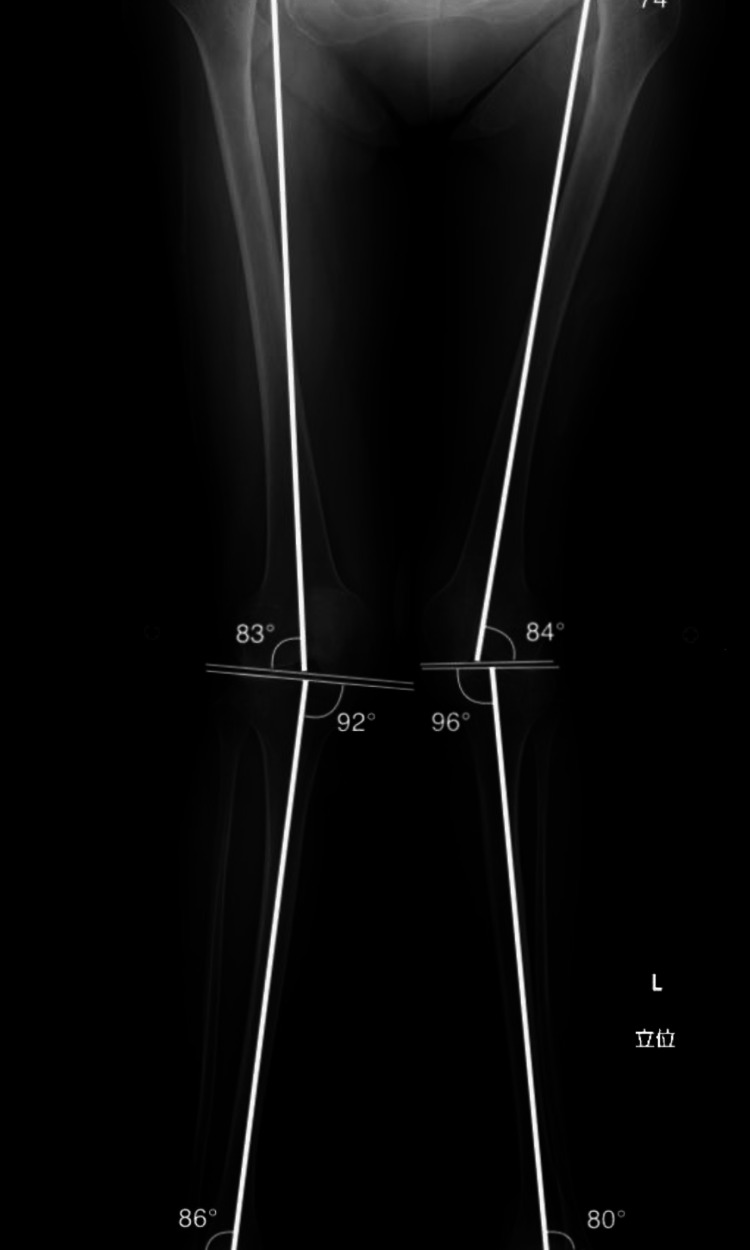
Preoperative measurement of alignment parameters Preoperative Radiograph of the entire lower limb with coronal alignment parameters.
